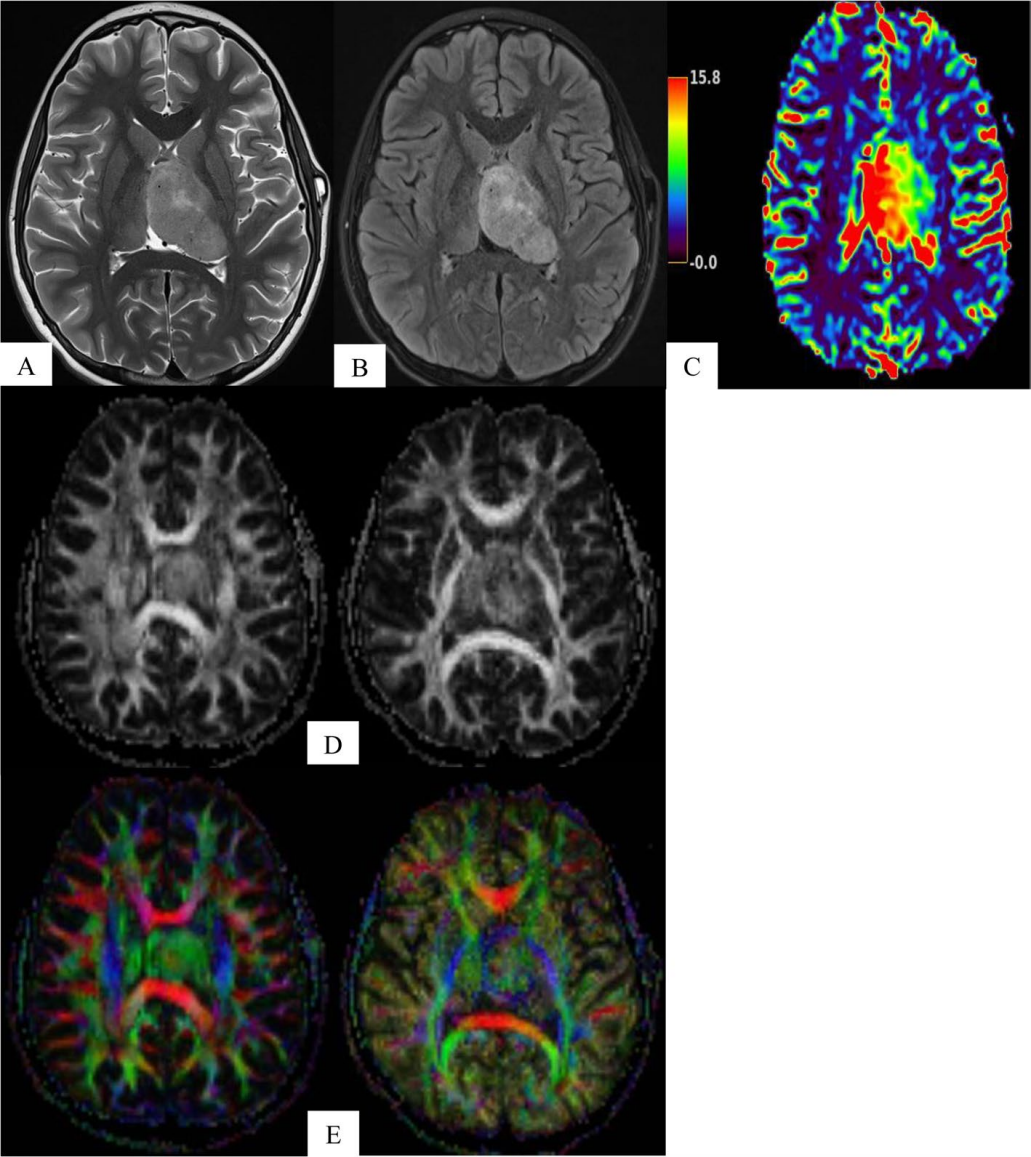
A-E 11-Year-Old Patient with Biopsy Proven Left Thalamic High-Grade Glioma with EGFRvIII Mutation. Axial T2-Weighted (A) and FLAIR MRI (B) show a large left thalamic mass with overall low signal intensity. MRI perfusion (C) shows elevated rCBV. Fractional anisotropy (D) and corresponding color-coded vector maps (E) show high signal
